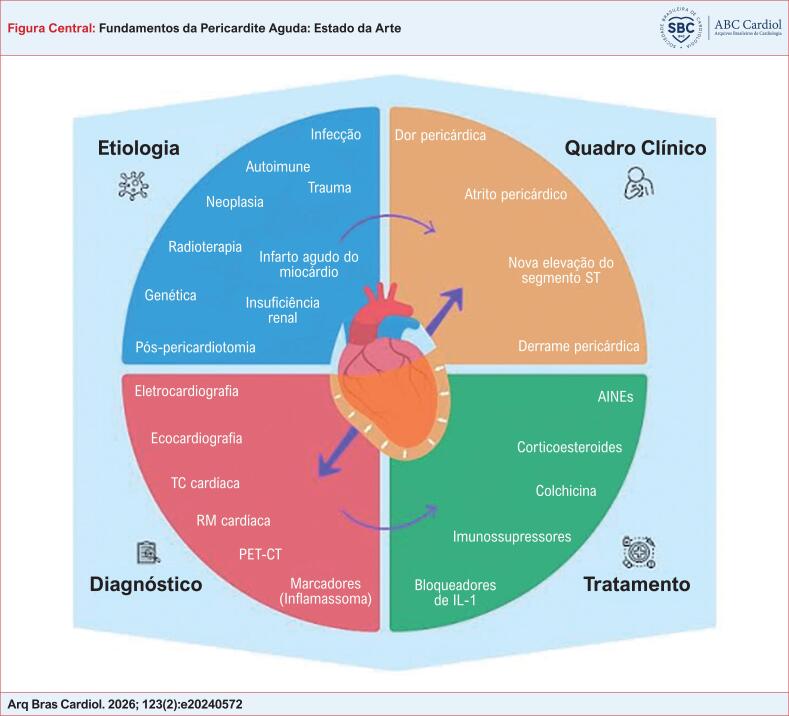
Mapa conceitual sobre etiologia, quadro clínico, diagnóstico e tratamento medicamentoso da pericardite aguda. AINEs: anti-inflamatórios não esteroidais; IL-1: interleucina-1; PET-CT: tomografia por emissão de pósitrons associada à tomografia computadorizada: RM: ressonância magnética; TC: tomografia computadorizada.
